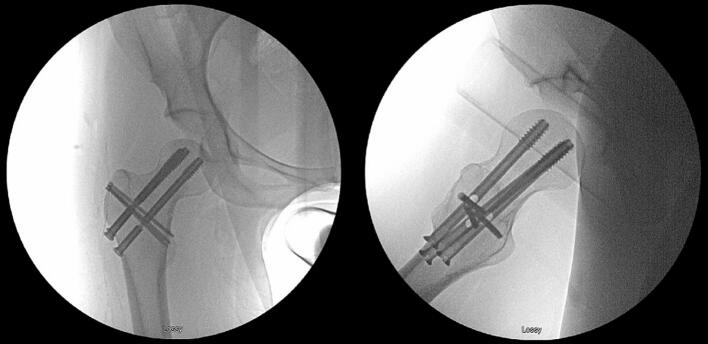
Intraoperative final AP and lateral radiographs following anatomic reduction and fixation of the displaced femoral neck fracture using the SimpliFix implant.
